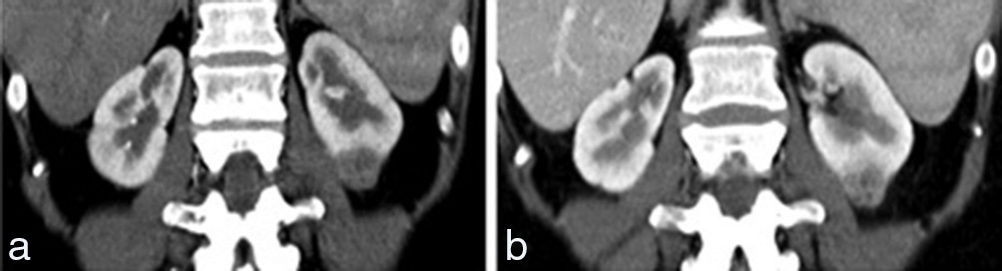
Coronal CT scan of a 31-year-old female with Crohn’s disease shows the left renal mass is homogeneously hypoenhancing in the arterial phase (a) and demonstrates patchy enhancement in the portal venous phase (b) with some hypodense internal foci.
